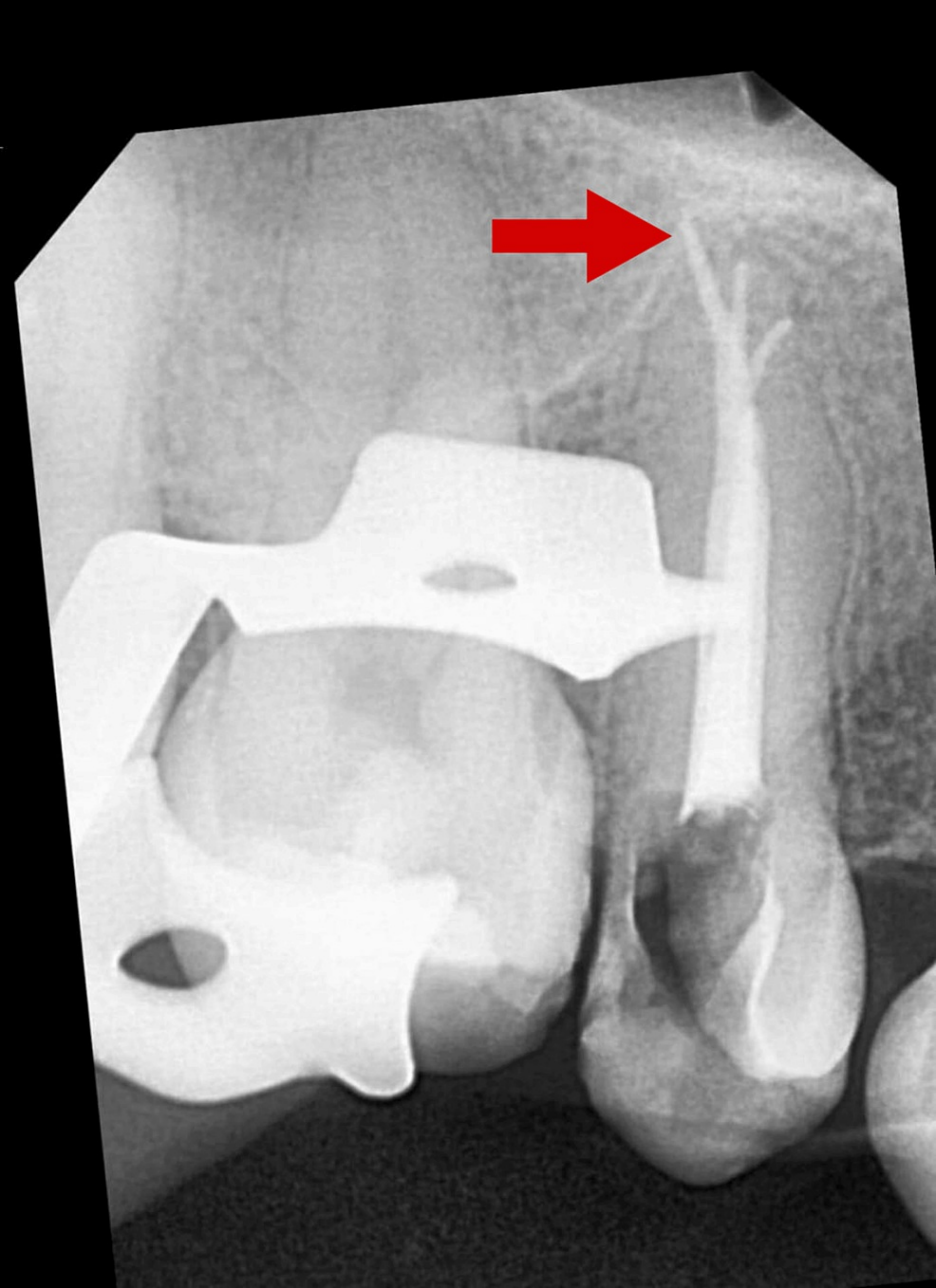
Obturation radiograph Arrow depicting distobuccal canal
